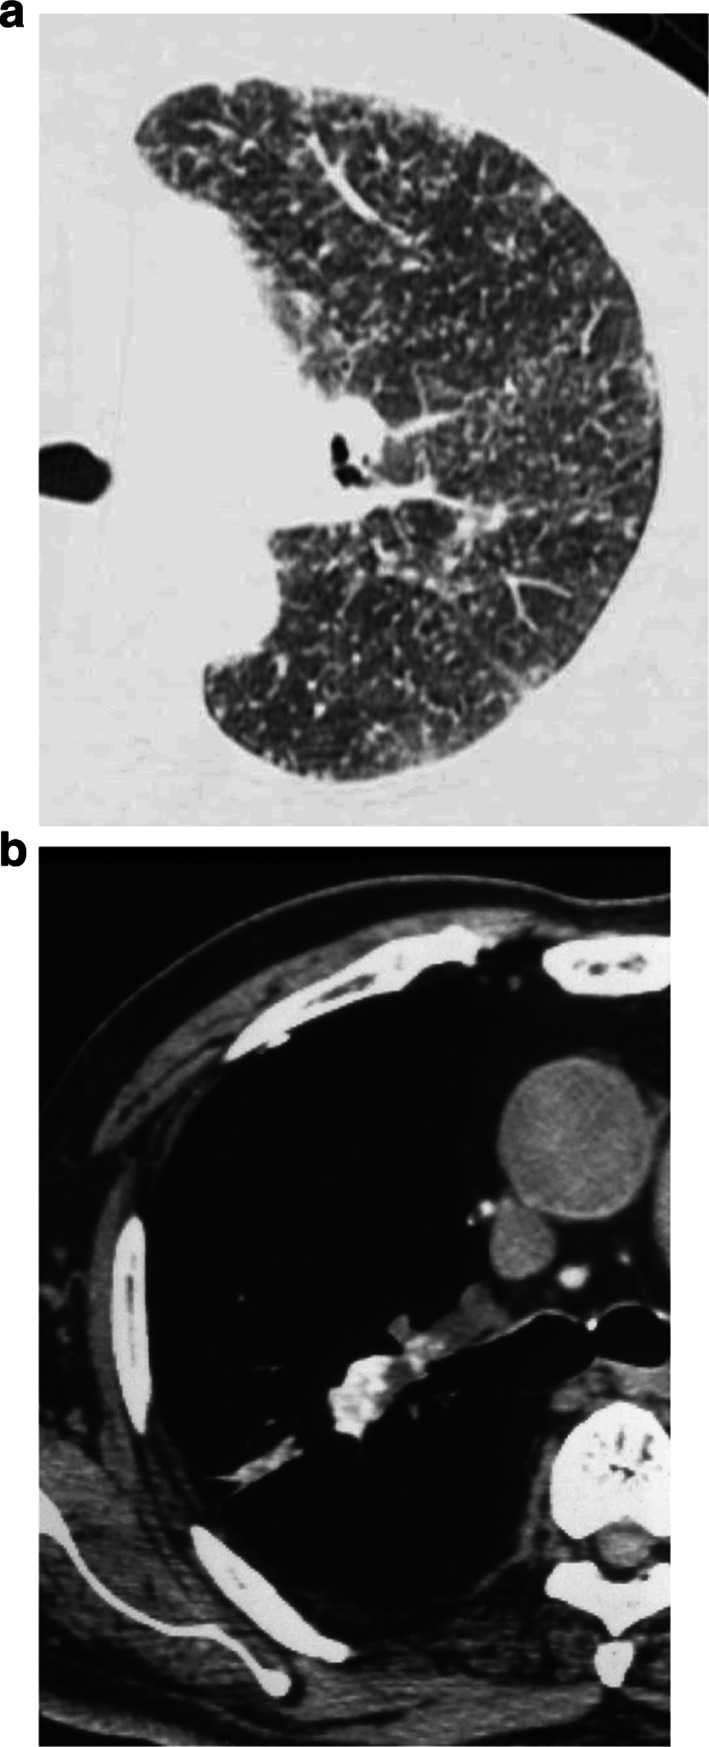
61-year-old man with inhalational talc pneumoconiosis employed in talc industry for 20 years. a HRCT scan shows diffusely distributed small nodules. They are separated from the pulmonary vein or the pleura at a distance of about 2–3 mm and are separated regularly from each other at a distance of about 2–3 mm. b CT scan at mediastinal setting of 61-year-old man with inhalational talc pneumoconiosis shows large opacity and lymph nodes containing high-attenuation material
